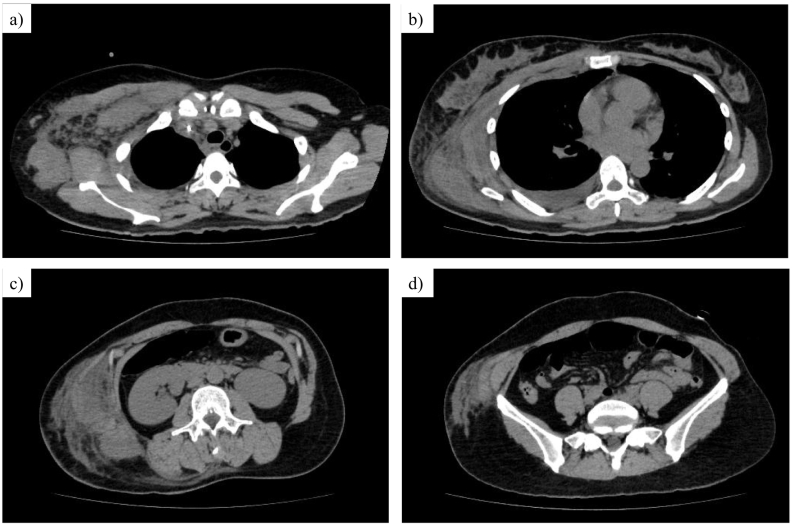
Computed tomography reveals diffuse soft tissue inflammation from the right axilla extending to the ipsilateral lower abdomen. It involved multiple lymphadenopathic areas in the axilla (a), a swollen mammary gland (b), and severe inflammation between oblique muscles (c, d).
